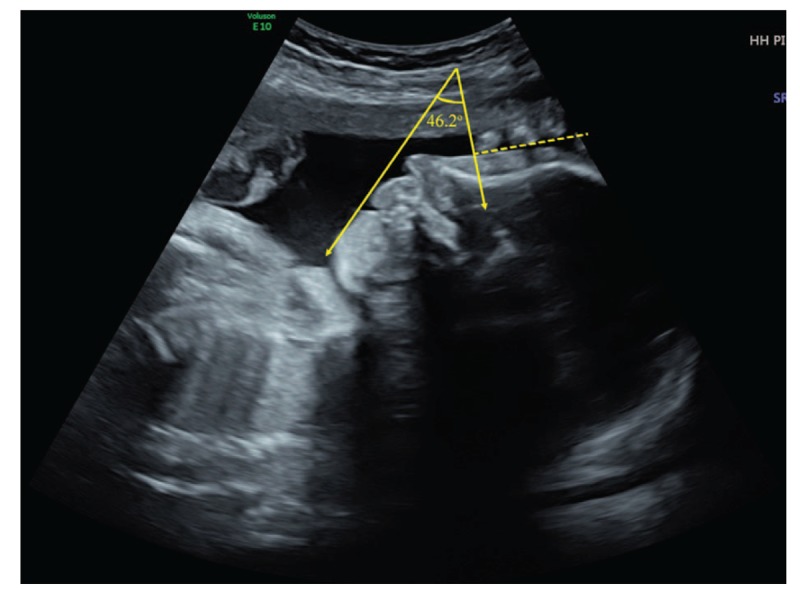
Two-dimensional ultrasound of the fetal facial profile with the IFA demonstrated. IFA = the inferior facial angle.
